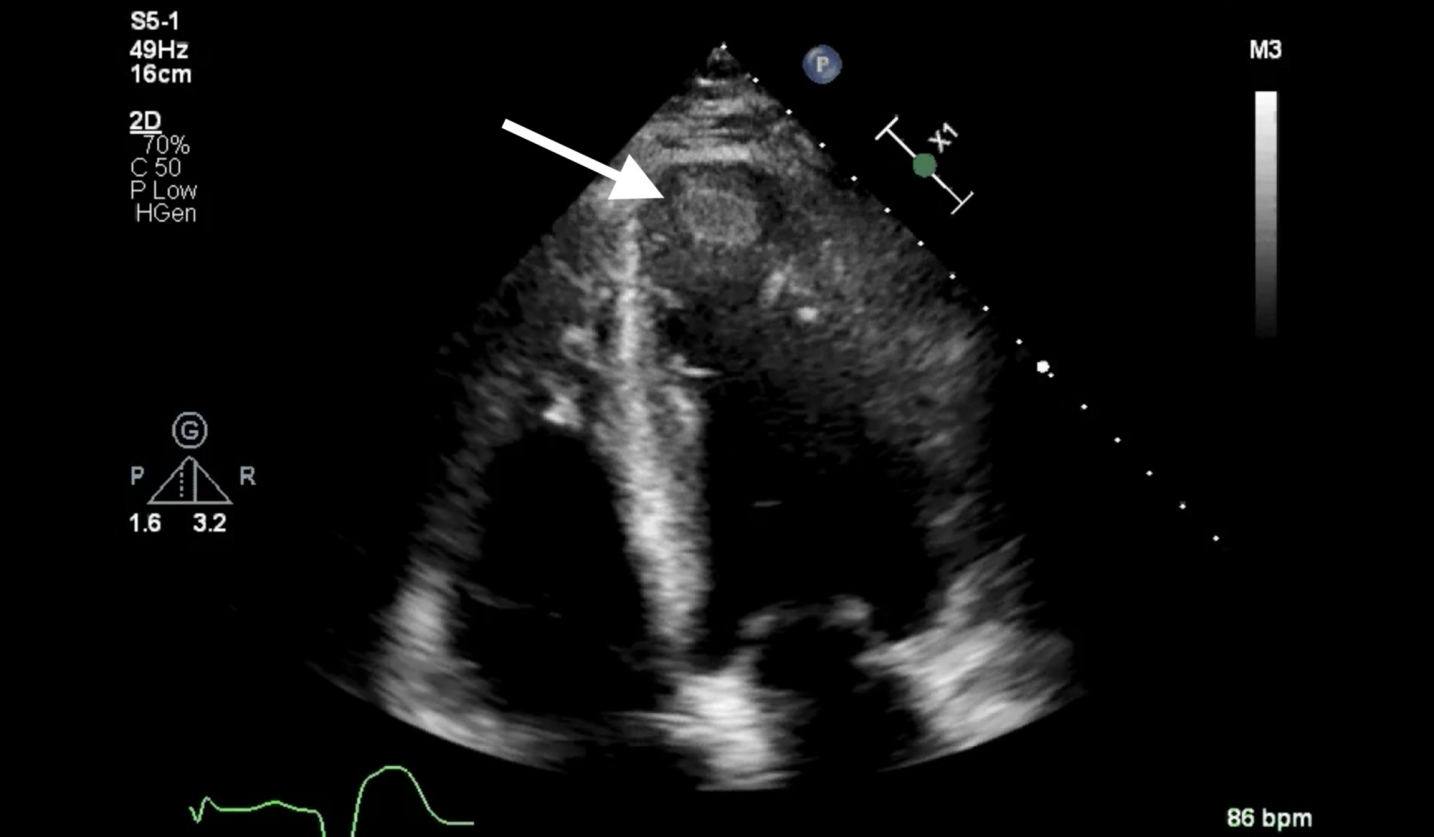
Echocardiographic findings of left ventricular apical thrombus
